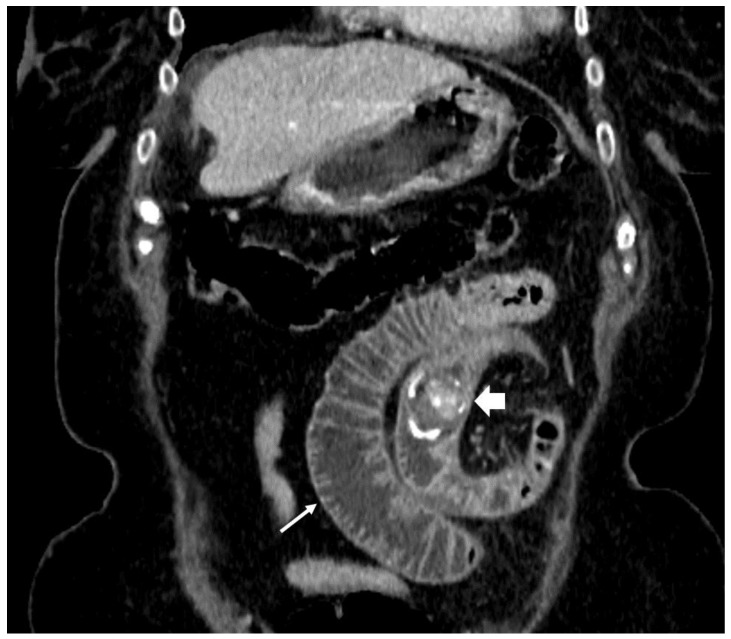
87-year-old female. Abdominal CT scan, coronal plane, portovenous phase. Dilated segment of proximal jejunal loops (thin arrow) caused by migrated gallstone (thick arrow) obstruction. Distal to the gallstone, jejunal loops are collapsed.
